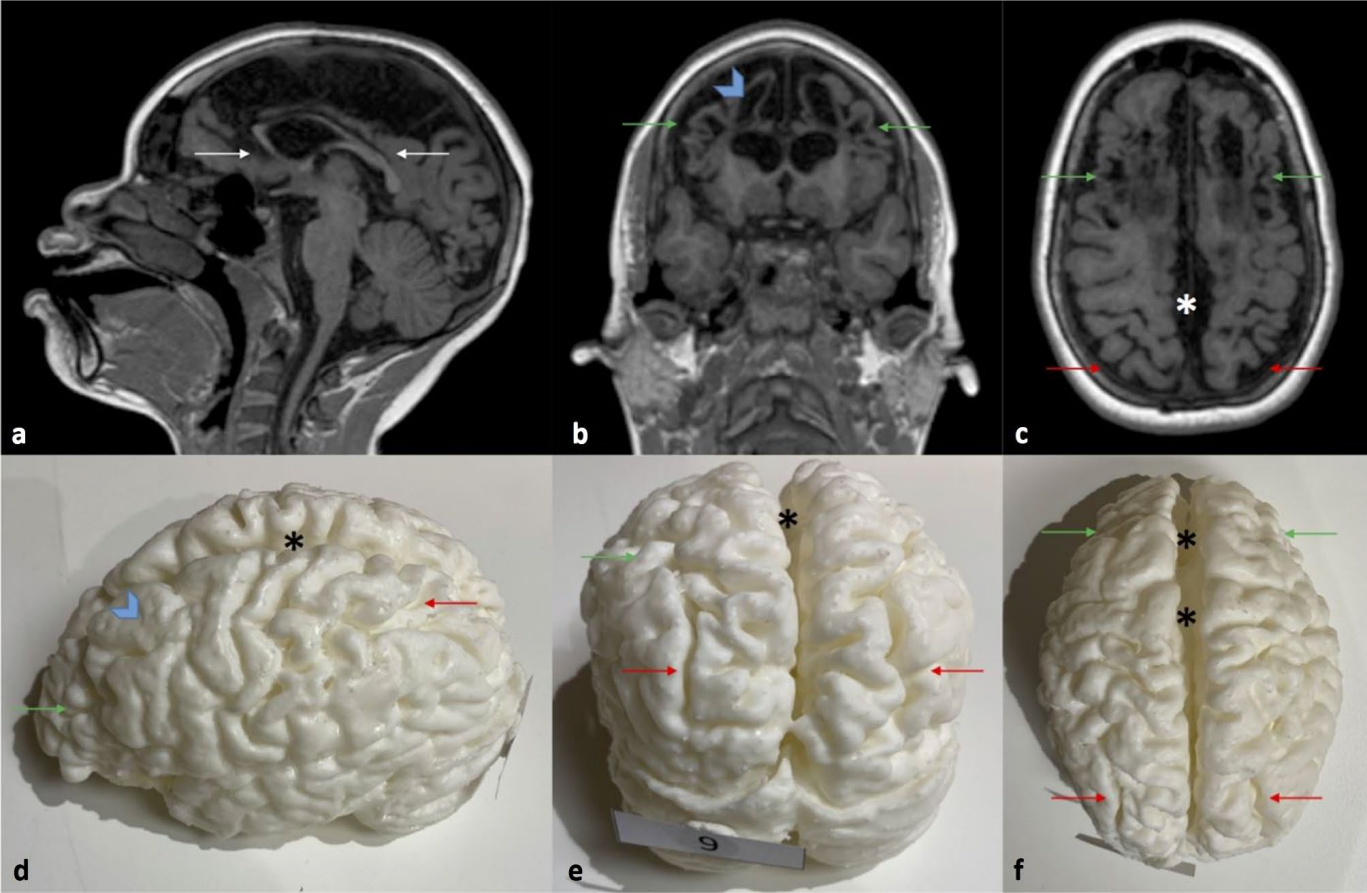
T1W MRI images in sagittal (a), coronal (b), and axial (c) planes with images of the corresponding 3D printed model in different views (d–f). This is a 10-year 6-month-old girl who suffered a remote prior hypoxic ischemic injury at term, with a combined partial prolonged and profound injury. The corpus callosum is diffusely thinned as a result of global atrophy (white arrows in (a)). The green arrows depict areas of focal cystic encephalomalacia and ulegyria within the anterior inter-arterial watershed of both cerebral hemispheres and the corresponding overlying cortical atrophy. The blue chevron depicts an area that required a manual patch (after automatic segmentation) to cover a defect due to the segmentation not recognizing the residual thin cortex overlying the cystic encephalomalacia. The red arrows depict atrophy with deep sulci of the posterior inter-arterial watershed bilateral. The asterisks (white and black) depict inter-hemispheric fissure widening due to the diffuse atrophy and associated lentiform separation of the hemispheres at the involved regions
